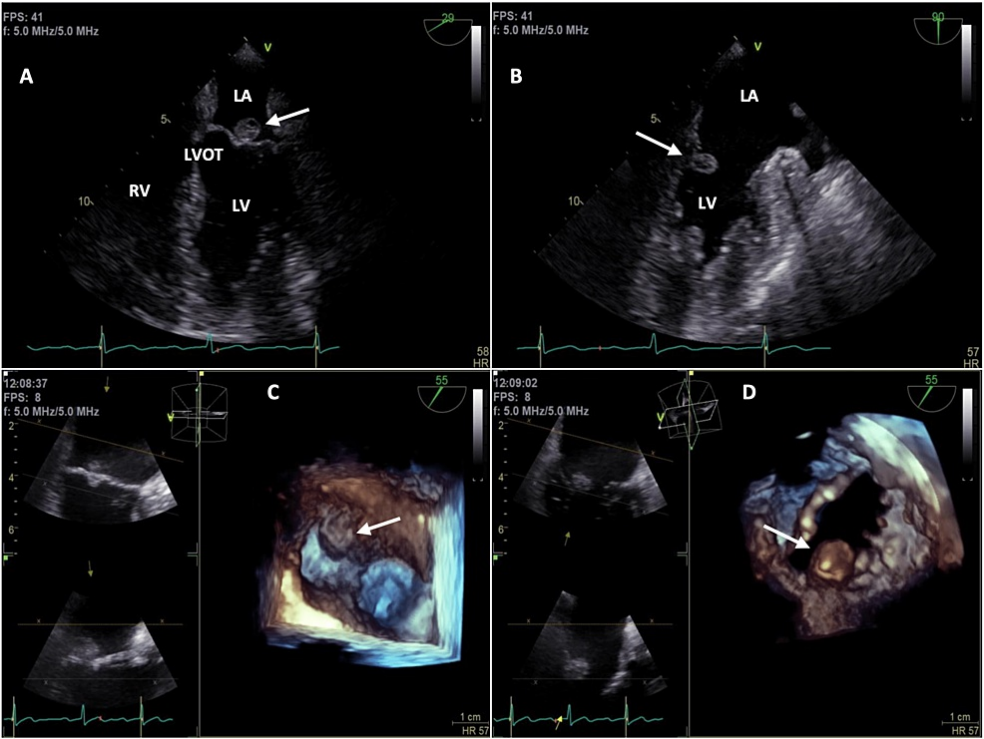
Transesophageal echocardiogram showing a 1.2×1.4 cm echogenic mass attached to the atrial side of the posterior mitral leaflet (white arrows). (A and B) Apical view during systole and diastole, respectively. (C and D) Three-dimensional view during systole and diastole, respectively. Note mass moving into the left ventricle during diastole (B and D). LA: left atrium; LV: left ventricle; LVOT: left ventricular outflow tract; RV: right ventricle
